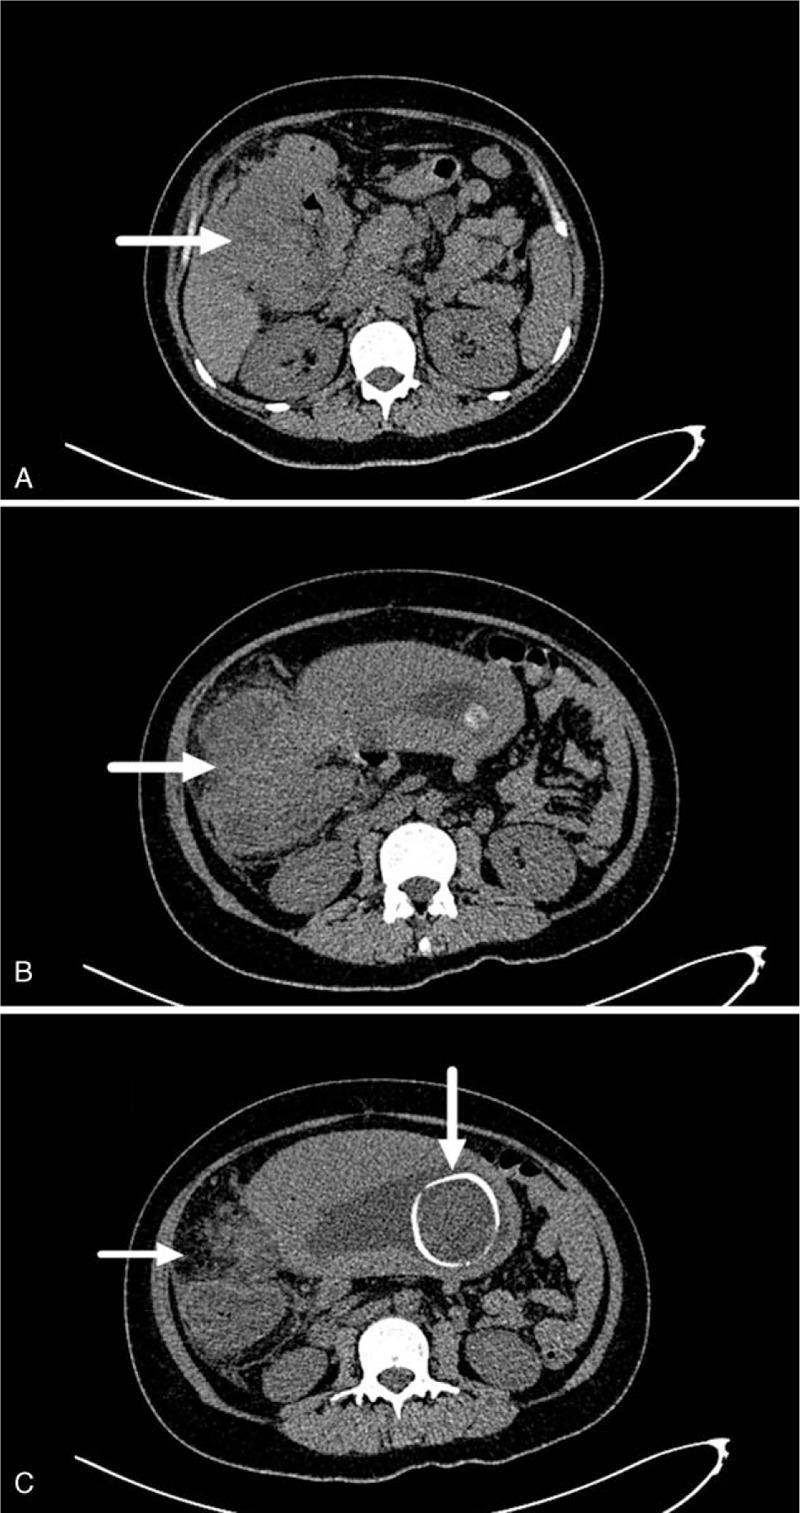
Computed tomography abdomen images. (A) Incrassation of the ascending colon wall and exudative change around it. (B) Tumor extended to ileocecus of the colon. (C) The skull of the fetus can be seen with similar diameter to the tumor.
